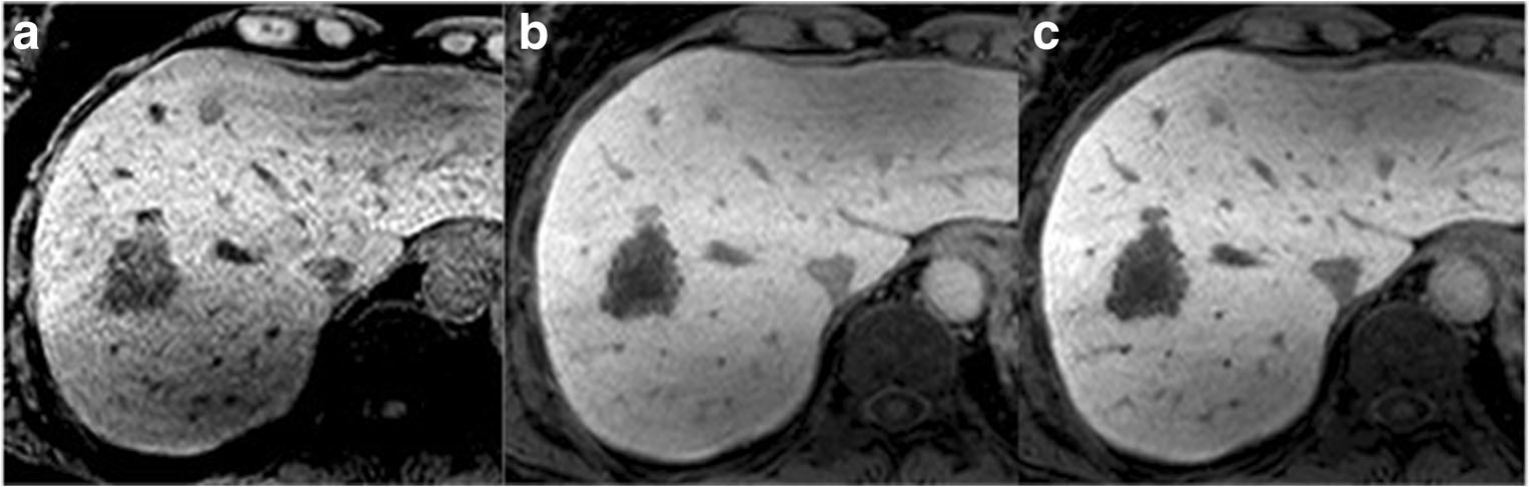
Pathology-confirmed colorectal liver metastases on preoperative 3D axial T1 MRI at (a) precontrast, (b) 10-min post-gadoxetate injection, and (c) 20-min post-gadoxetate injection in a 69-year-old male demonstrating weak tumour enhancement
